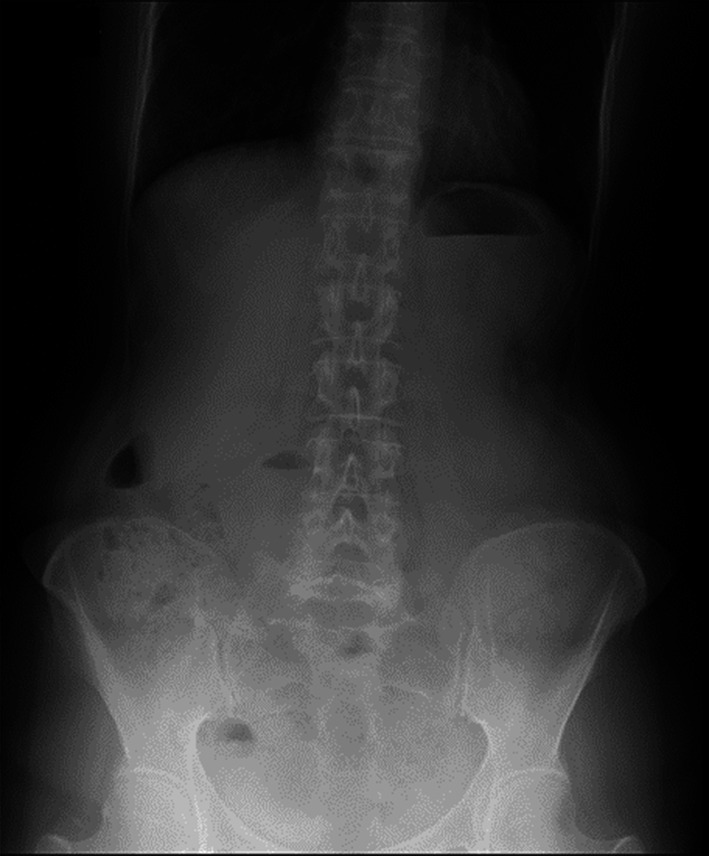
Abdominal radiographs on admission showed no evidence of bowel obstruction
